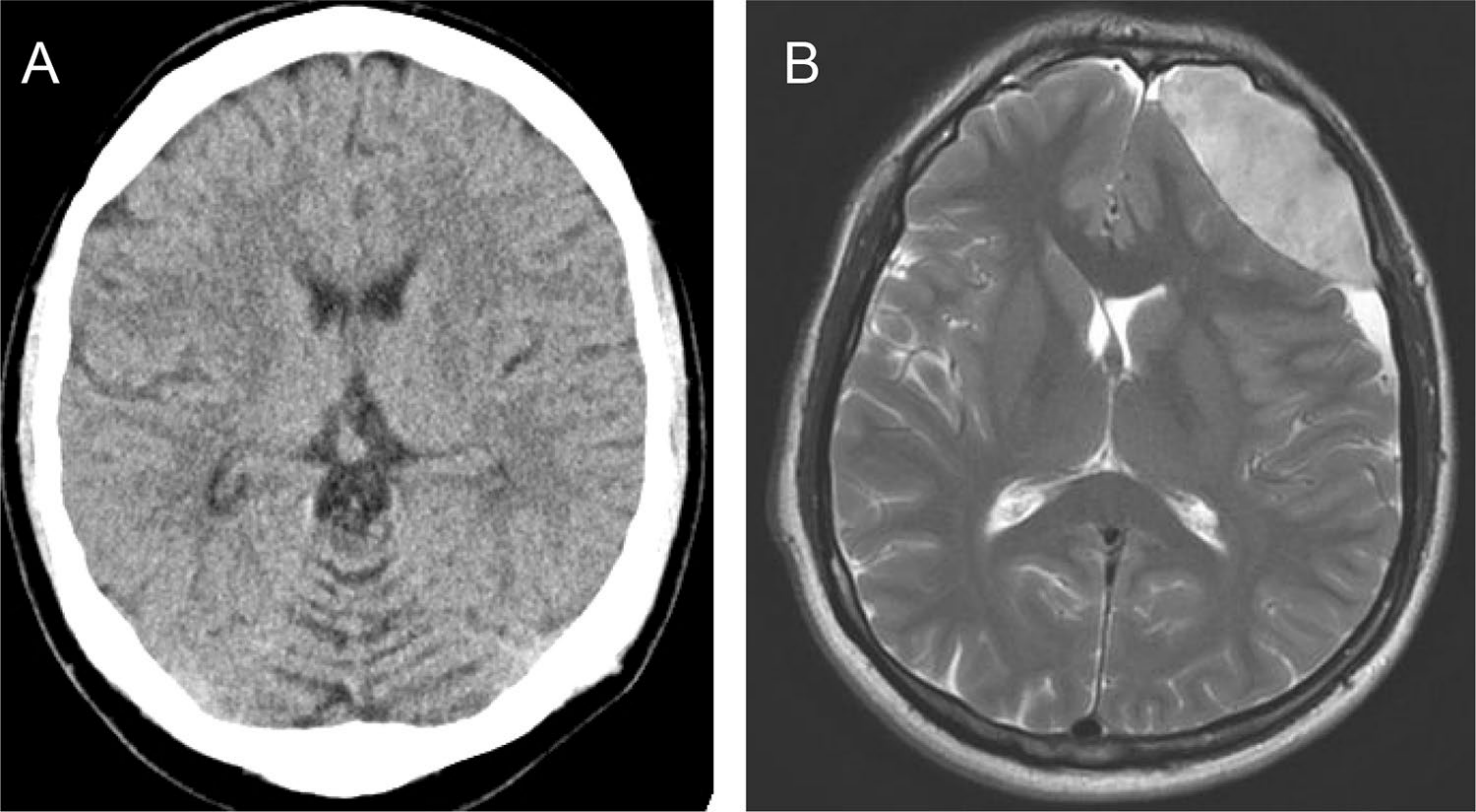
a Normal cranial computed tomography in a 32-year-old man on admission due to a headache and neck pain. b T_2_-weighted MRI revealing pronounced frontal epidural hematoma (7.5 × 2.5 × 5 cm) with consecutive midline shift of 10 mm following lumbar puncture. (Courtesy of Hans-Peter Dinkel, MD, PhD, Radiological Institute, Municipal Hospital Landshut)
